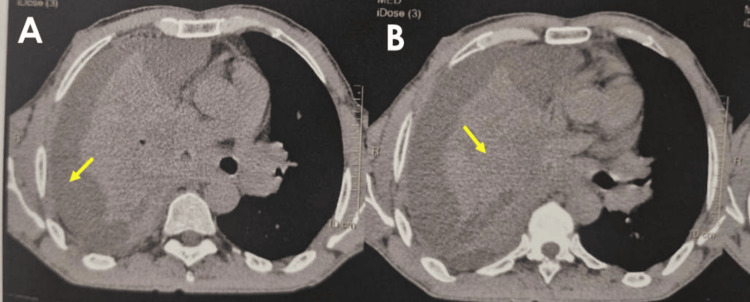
Axial chest CT images demonstrating right pleural effusion with associated atelectasis (A) Large right pleural effusion (arrow).
